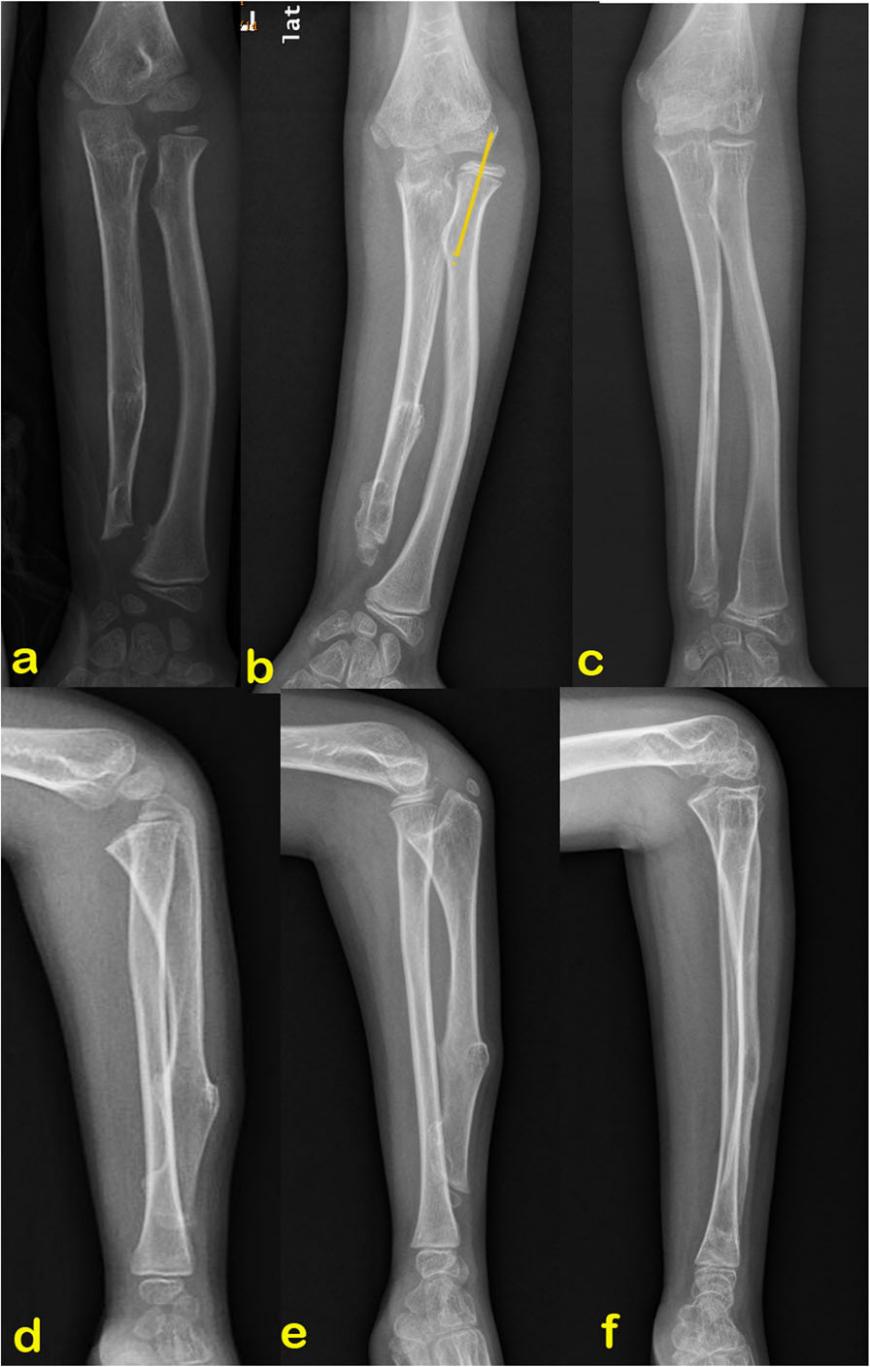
a, b Forearm deformity progressed with age, radial head proximal migrant and the radial neck axis lateral deviation were found 3 years later after the first clinical assessment, and ulnar gradual lengthening with osteochondroma resection was performed at a 10-year-old. d, e Lateral radiograph of the same patient. c, f 2.1 years after the external fixator was removed, both the proximal radioulnar joint and the radiocapitella joint were well maintained
